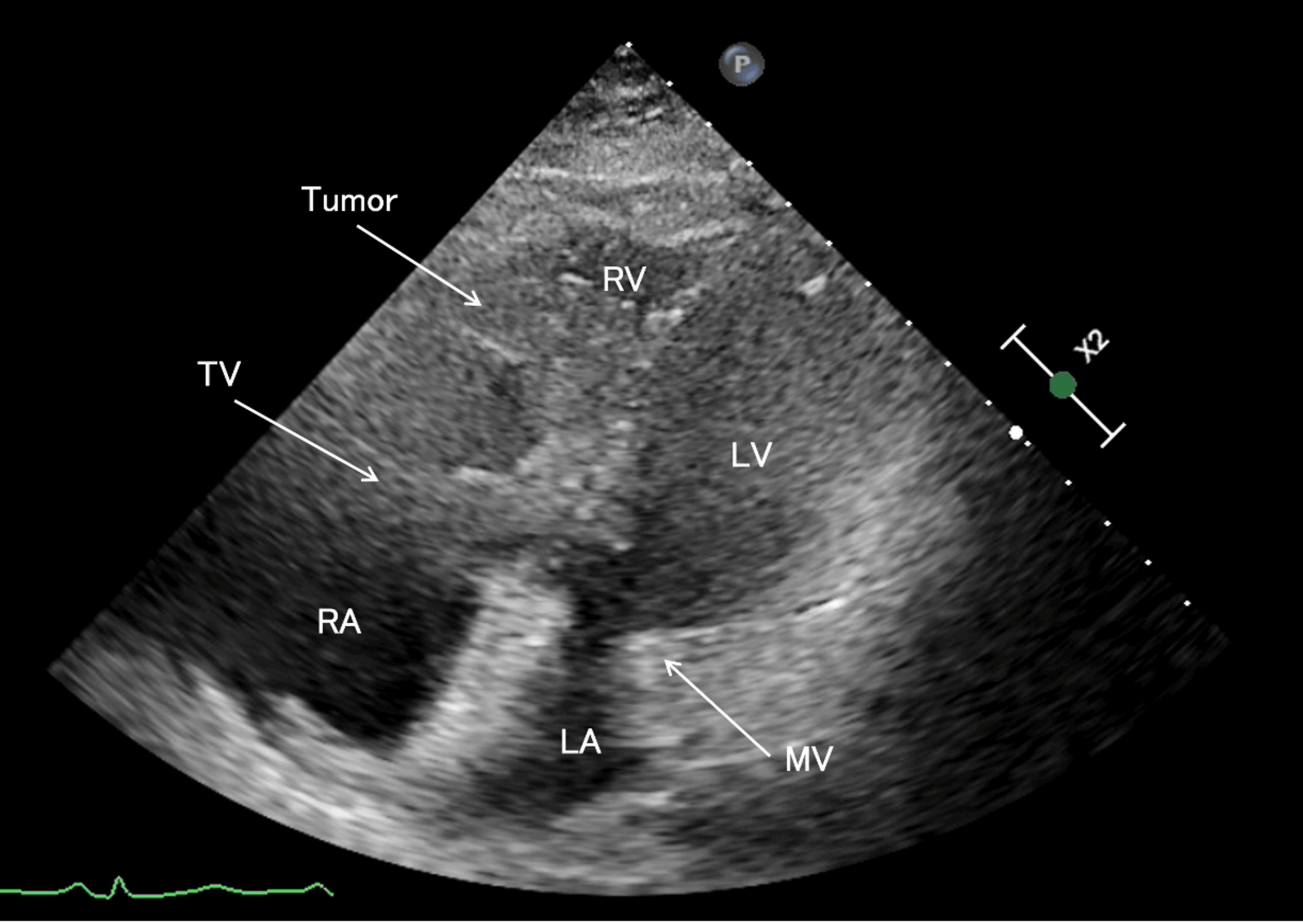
Transthoracic echocardiography, four-chamber view upon arrival A giant tumor originating from the inferior vena cava is extended into the right ventricle, becoming entrapped in the tricuspid valve during diastole. A mass was observed moving between the right atrium and right ventricle in synchrony with cardiac contractions across the tricuspid valve. The right ventricular contractility appeared visually preserved, but the left ventricle demonstrated a D-shaped deformity. The mass was partially cystic and appeared as a spherical, isoechoic structure. TV, tricuspid valve; RA, right atrium; RV, right ventricle; MV, mitral valve; LA, left atrium; LV, left ventricle
